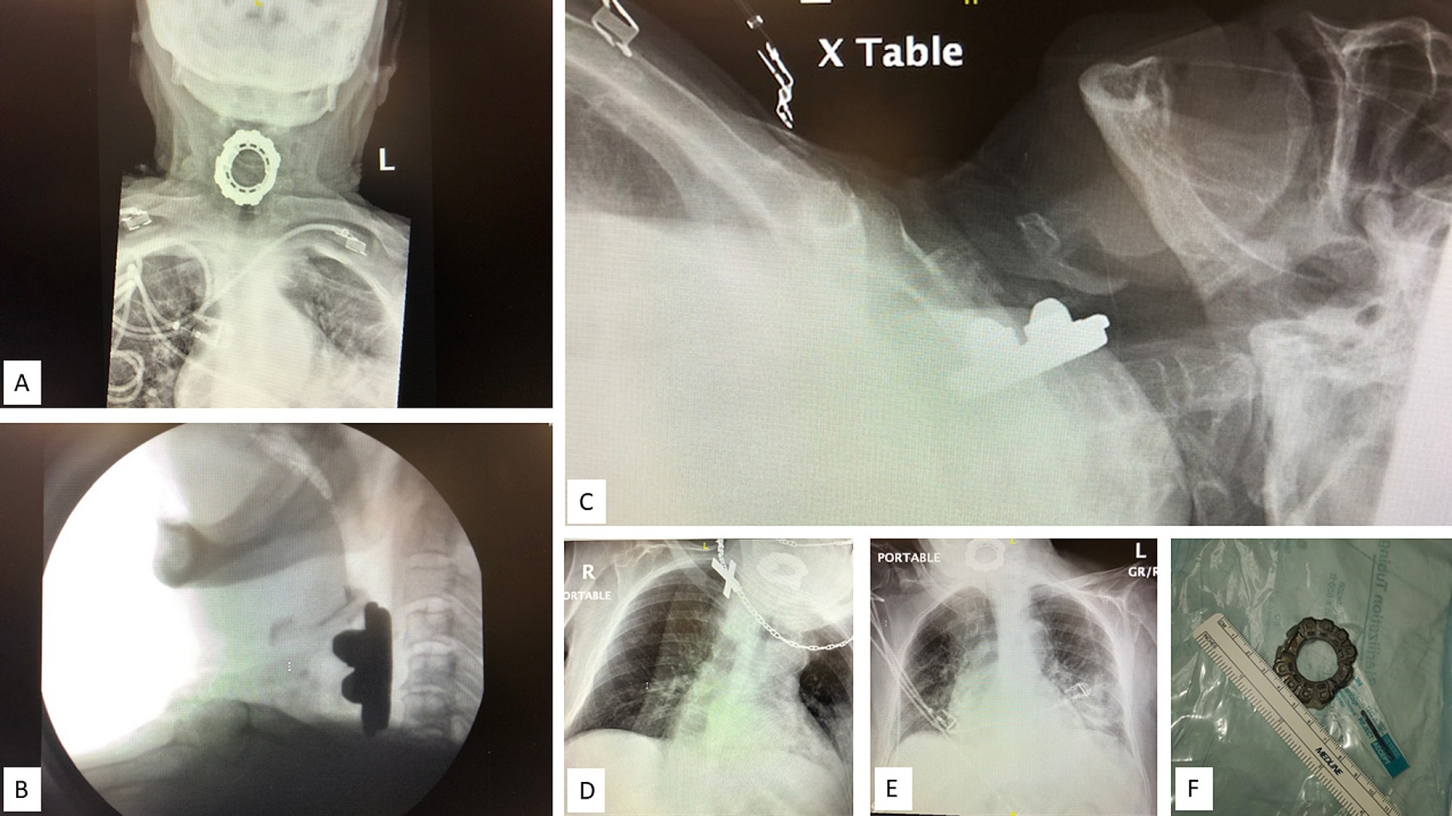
Comparative Chest and Neck Radiographs With Foreign Body in Place vs Extracted (A) Anteroposterior chest radiograph; (B) upright lateral neck radiograph; (C) supine lateral neck radiograph; (D) wide angle anteroposterior neck radiograph; (E) wide angle anteroposterior neck radiograph; (F) 3 cm diameter foreign body extracted (courtesy of Dr. David Gerth).
